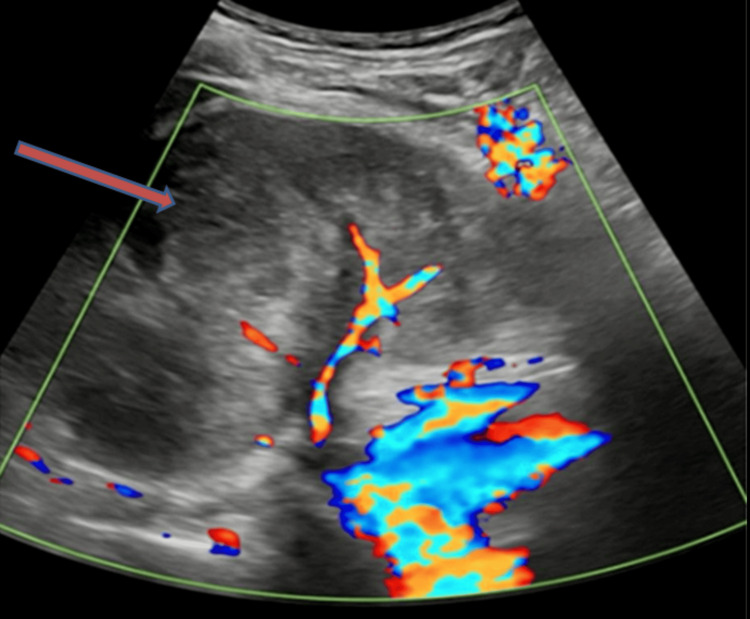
The color Doppler ultrasound image reveals a lack of significant color uptake within the lesion, while the surrounding renal parenchyma shows normal blood flow. The absence of color uptake suggests the lesion is avascular or has very low blood flow, characteristic of collecting duct carcinoma. This lack of vascularity can indicate necrosis or poor perfusion, associated with aggressive malignancies.
